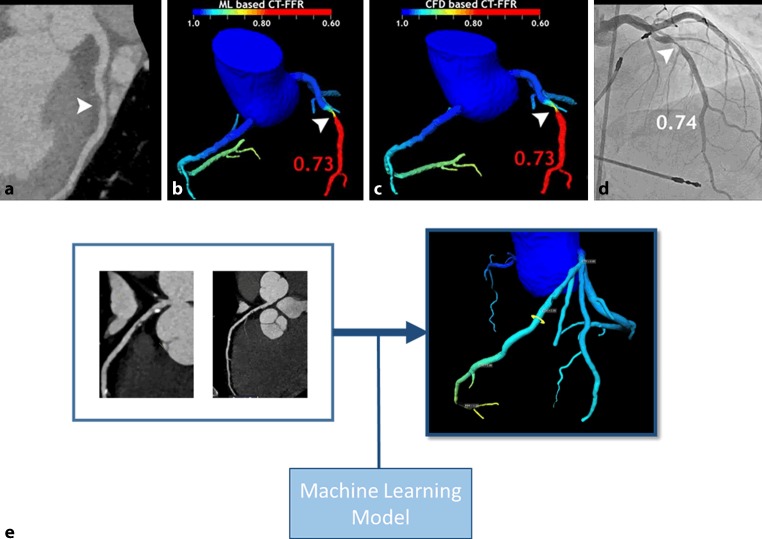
a–e A stenosis (indicated by the arrowheads) displayed in different imaging methods. a computed tomography coronary angiography (CTCA). b Calculation of fractional flow reserve (FFR) with a machine learning (*ML*) model. c Calculation of FFR with computational fluid dynamics (*CFD*). d Measurement of the stenosis during invasive coronary angiography. e From coronary CTCA to an AI-based 3D model of the coronary tree, displaying the FFR at different locations along the coronary arteries. (Images reproduced with permission [15])
